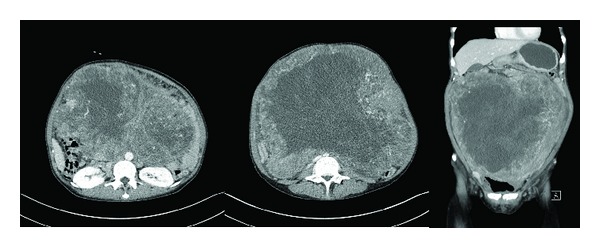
Abdominal computed tomography (CT) showing a huge solid mass occupying whole pelvis and abdomen.
